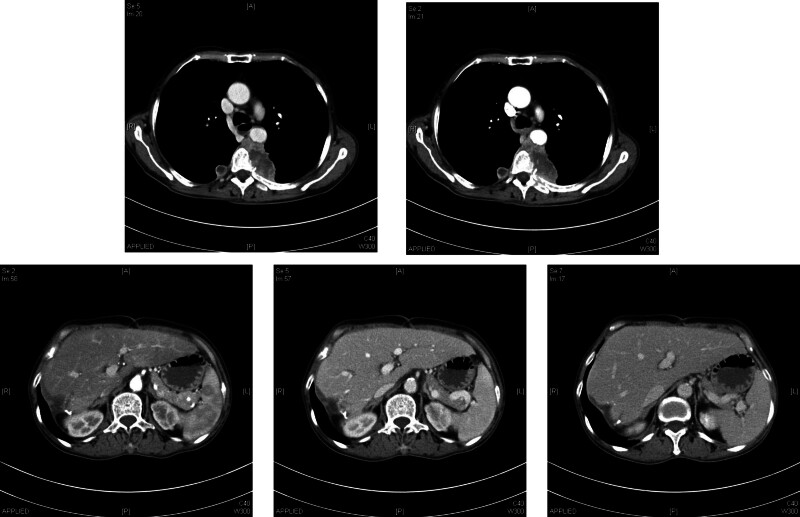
Chest and abdominal CT examination at 3 months postsurgery.
